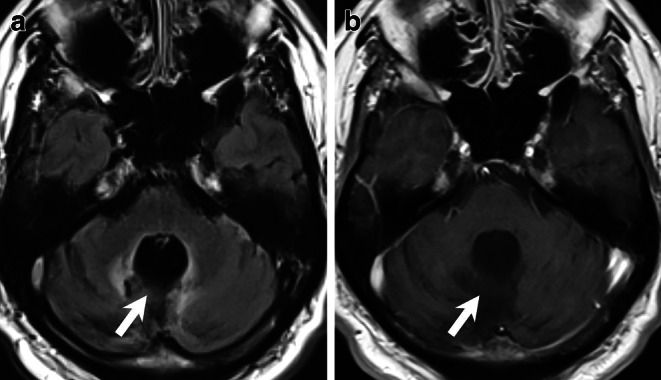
Bi-annual MRI follow-up from 2022. Axial FLAIR images (a), and axial T1-weighted images acquired after Gd administration consistently confirmed the complete removal of the tumour, with no evidence of recurrence at the site of the resection (a,b, *arrow*)
